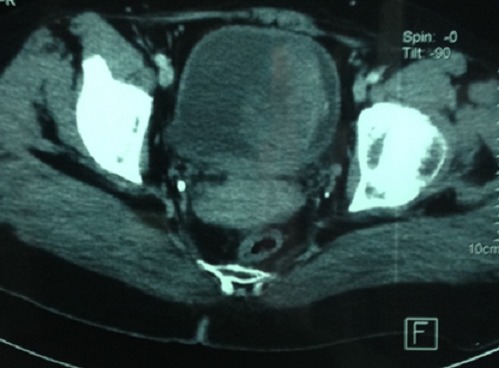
Axial image from a contrast abdominal CT in soft tissue windows demonstrates a homogeneous, well-circumscribed, solid mass on the left anterolateral bladder wall
